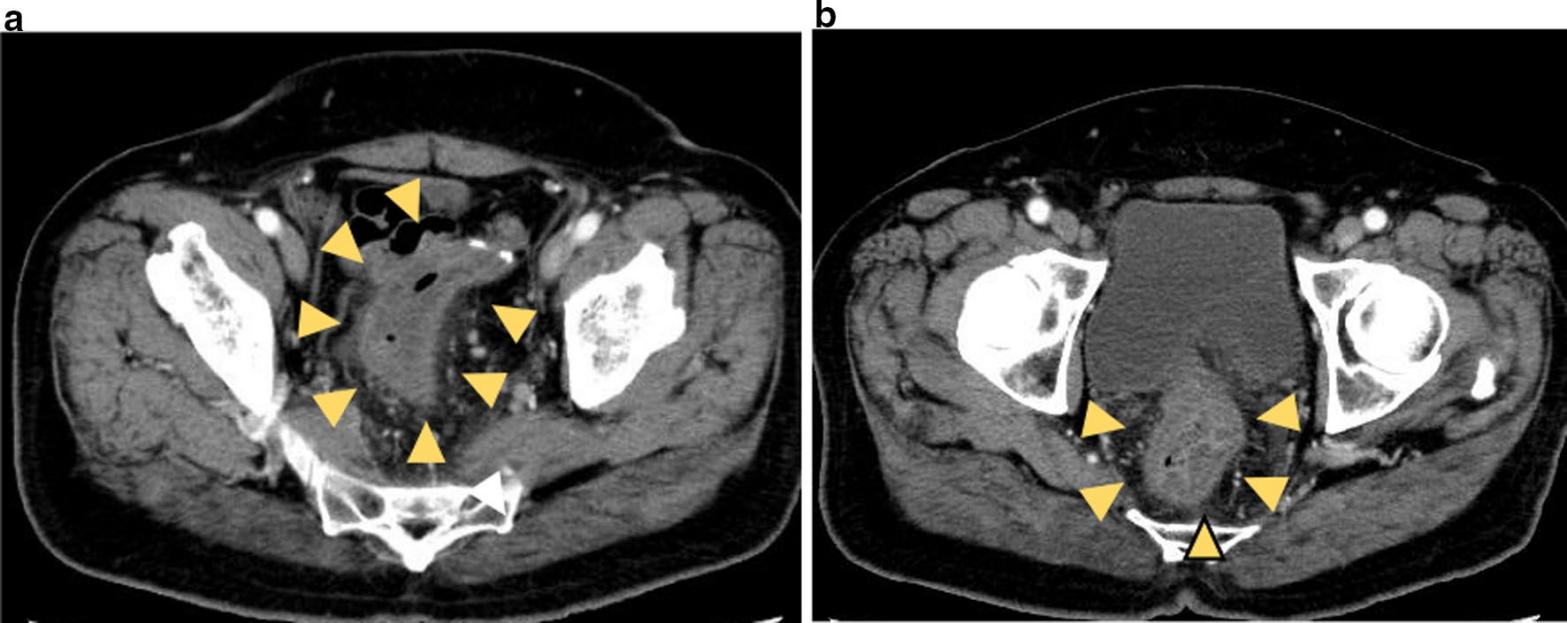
Abdominal computed tomography findings. The images show edematous changes in the anal side of the anastomosis (yellow arrow). a The severest part of the ischemic lesion is not located adjacent to, but b is located at a short distance from the anastomosis towards the anal canal
